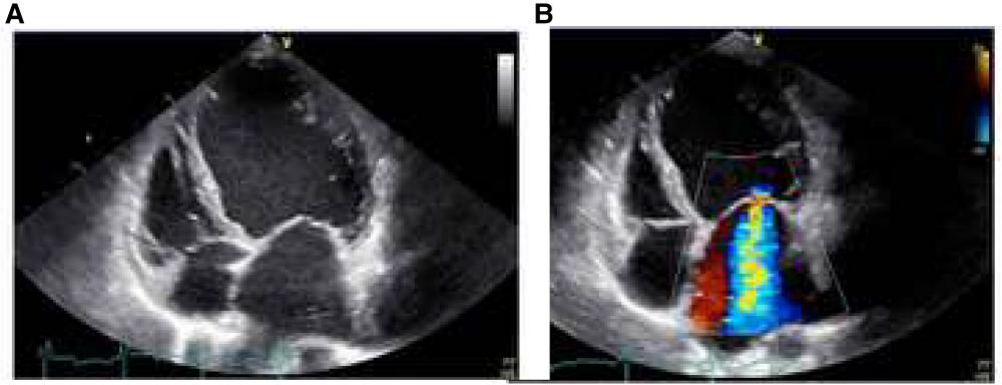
Echocardiogram. Four chamber view with severely dilated left ventricle (*A*) and moderate to severe mitral regurgitation (*B*).
